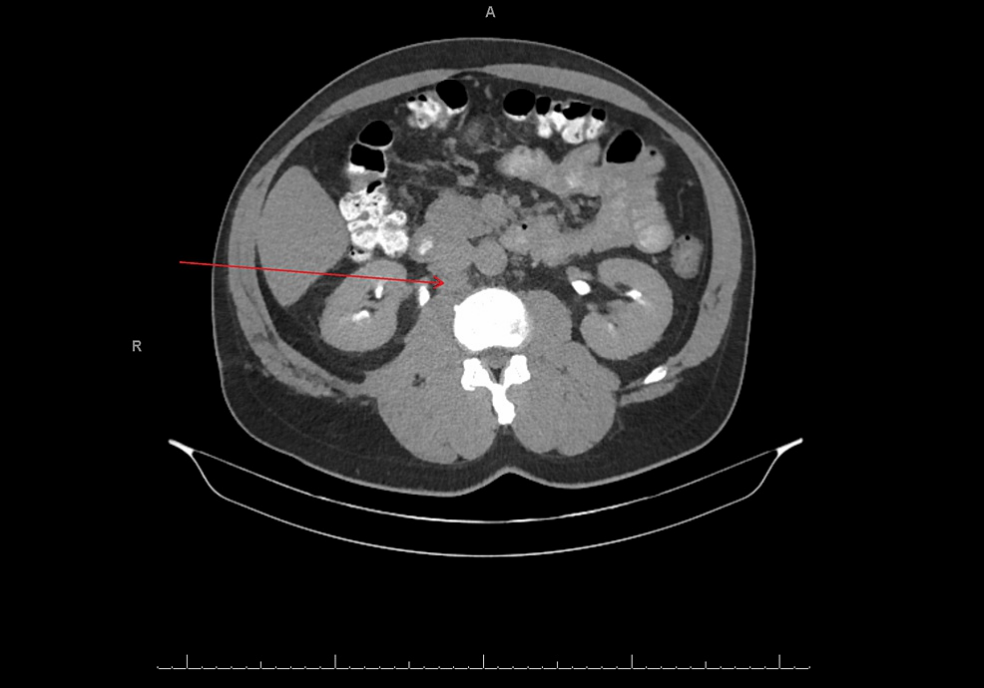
Pre-chemotherapy CT abdomen/pelvis with contrast showing retroperitoneal lymphadenopathy Arrow pointing at enlarged para-aortic lymph node.
